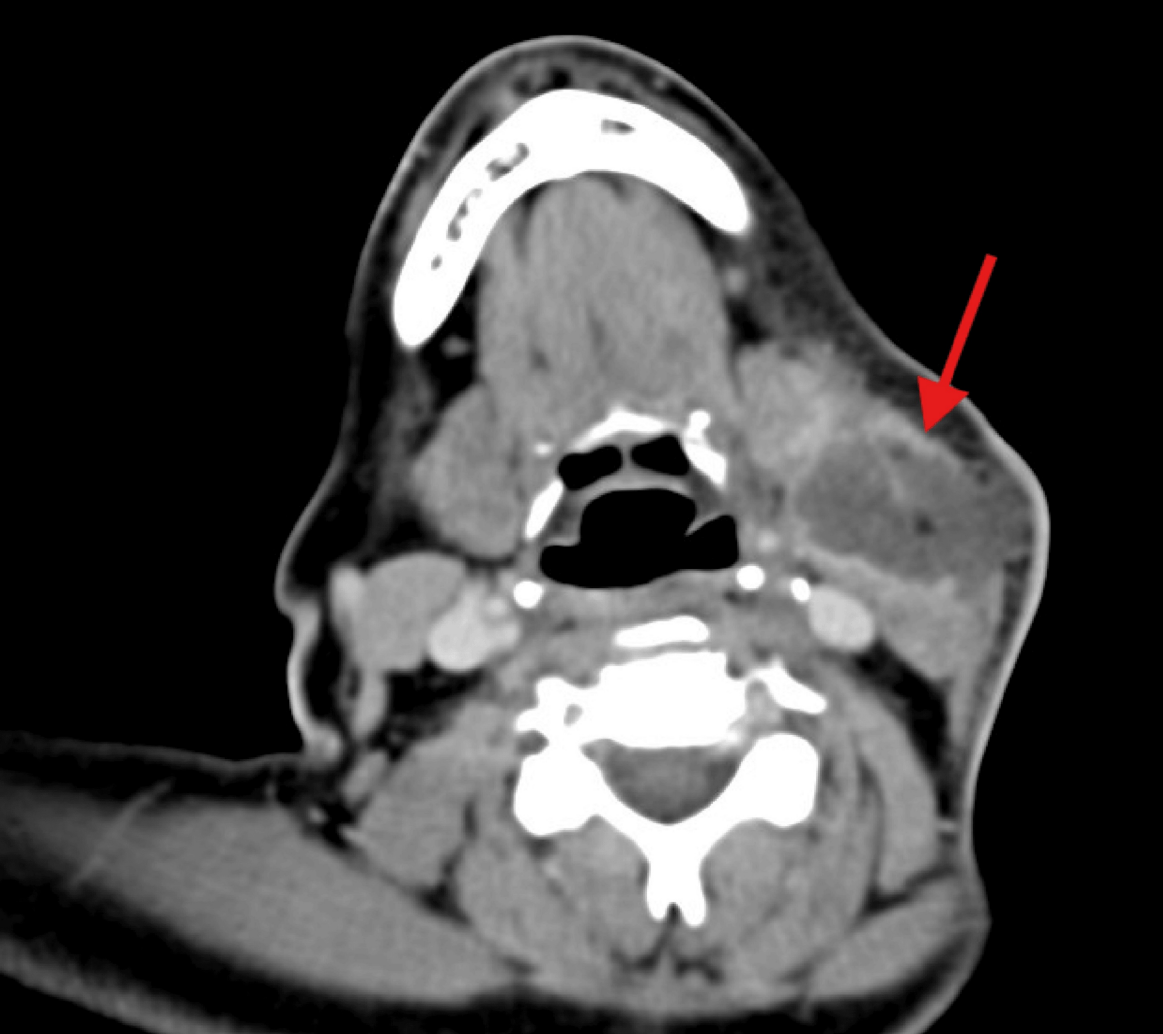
CT image showing Salmonella abscess of the left side of the neck at the suprahyoid and infrahyoid level CT: computed tomography
